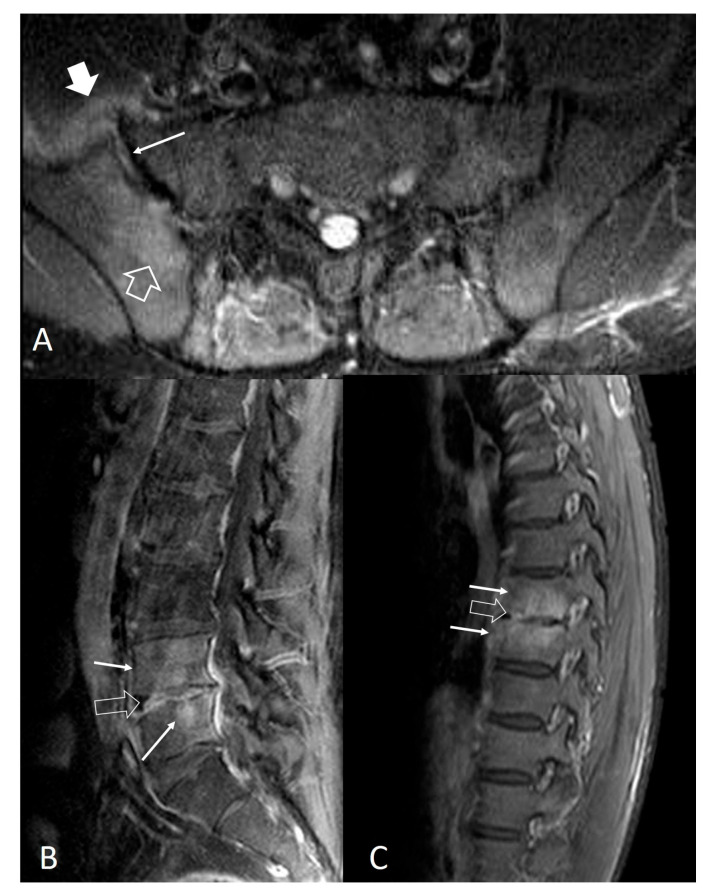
Noncontiguous multifocal musculoskeletal brucellosis. (A) Axial STIR MR image, showing bone marrow edema (open arrow), joint effusion (thin arrow), and capsular thickening (thick arrow) in keeping with sacroiliac joint involvement. Sagittal fat-suppressed contrast-enhanced MR images of the lumbar spine (B) and thoracic spine (C) showing discitis (open arrows) with spondylitis (thin white arrows).
